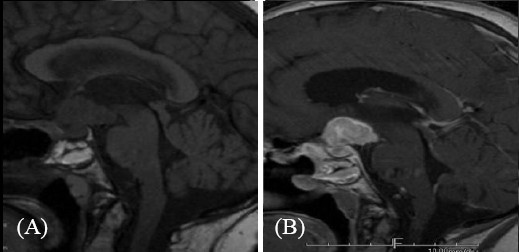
T1-weighted magnetic resonance imaging. (A) Pre-contrast mid-sagittal image of pituitary metastasis; (B) post-contrast detail of pituitary metastasis.
